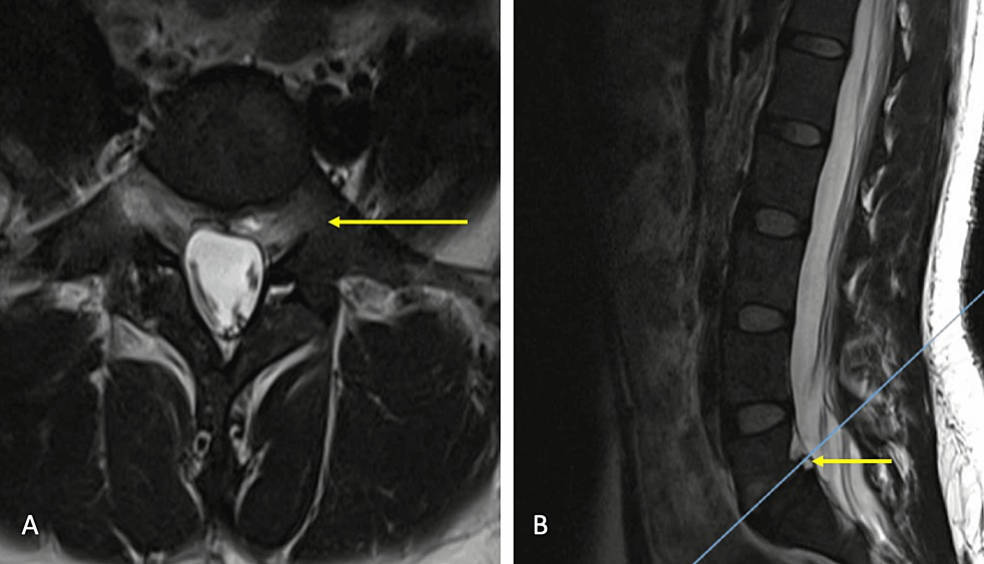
Preoperative imaging studies (A) Axial T2 MRI L5-S1 sequence without lumbar spinal stenosis, due to lytic spondylolisthesis (yellow arrow). (B) Sagittal T2 MRI L5-S1 sequence with grade 1 spondylolisthesis (yellow arrow). MRI: magnetic resonance imaging
